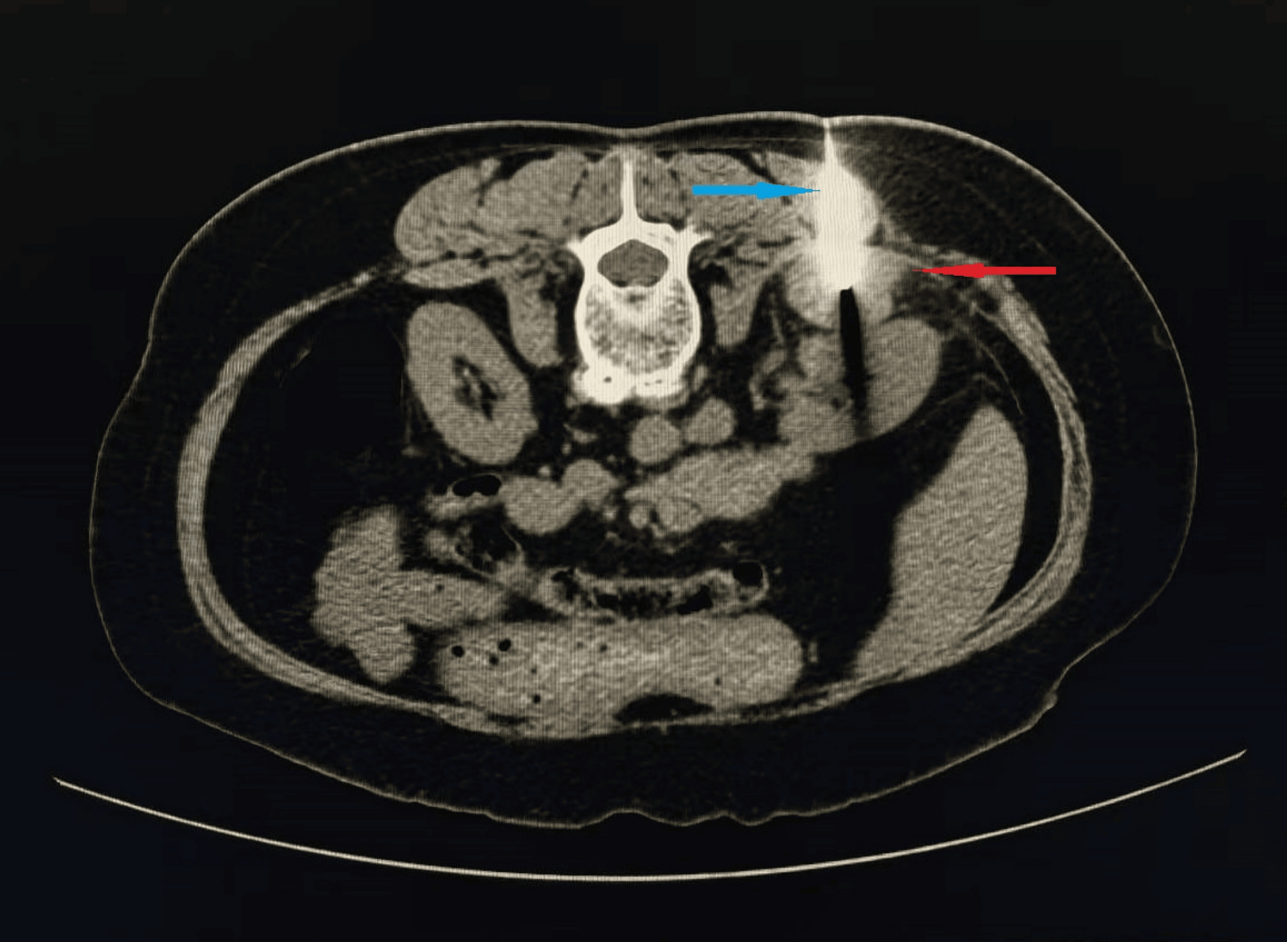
Image of a CT-guided percutaneous adrenal mass biopsy. The red arrow indicates the biopsied mass, and the blue arrow indicates the biopsy needle.
